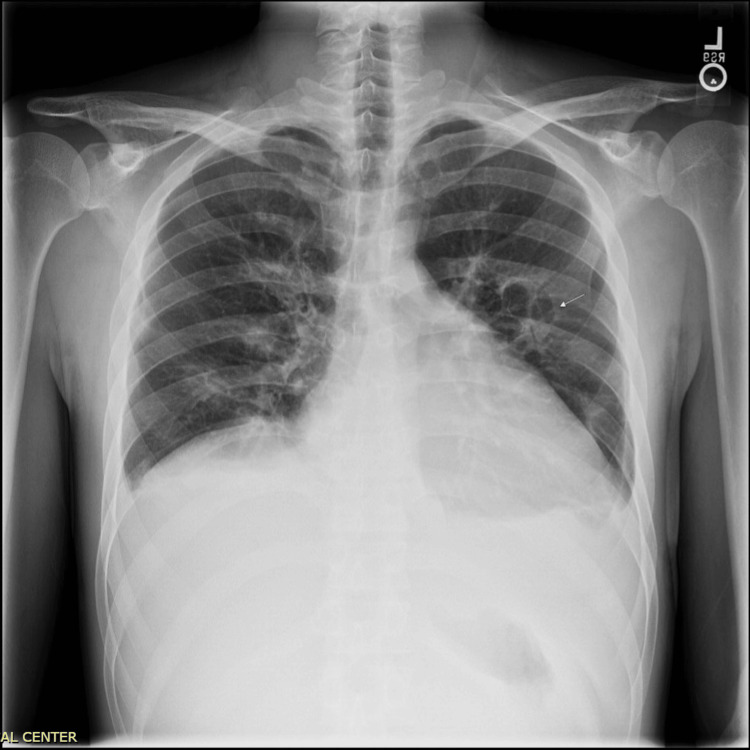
Chest radiograph demonstrating cardiomegaly with pulmonary venous congestion and bilateral interstitial opacities consistent with pulmonary edema.
